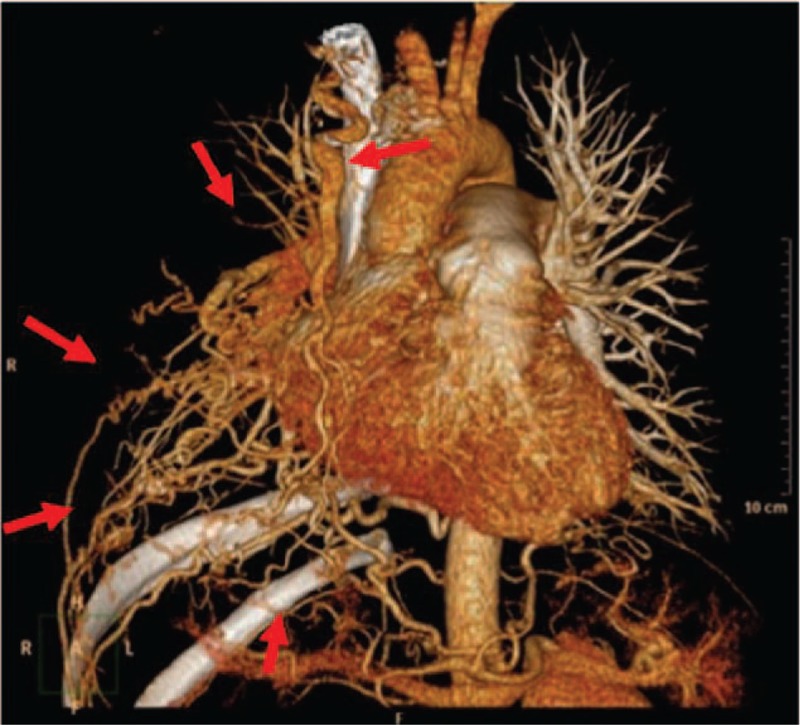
CT pulmonary angiography demonstrating that compared to the left pulmonary vessels, the right pulmonary arteries, intercostal arteries, and internal mammary artery were tortuous and dilated. Red arrows on the right side of the ascending aorta point to the internal mammary artery, and intercostal arteries are parallel to the ribs. Area around the red arrows is the range of the fistulae. CT = computed tomography.
